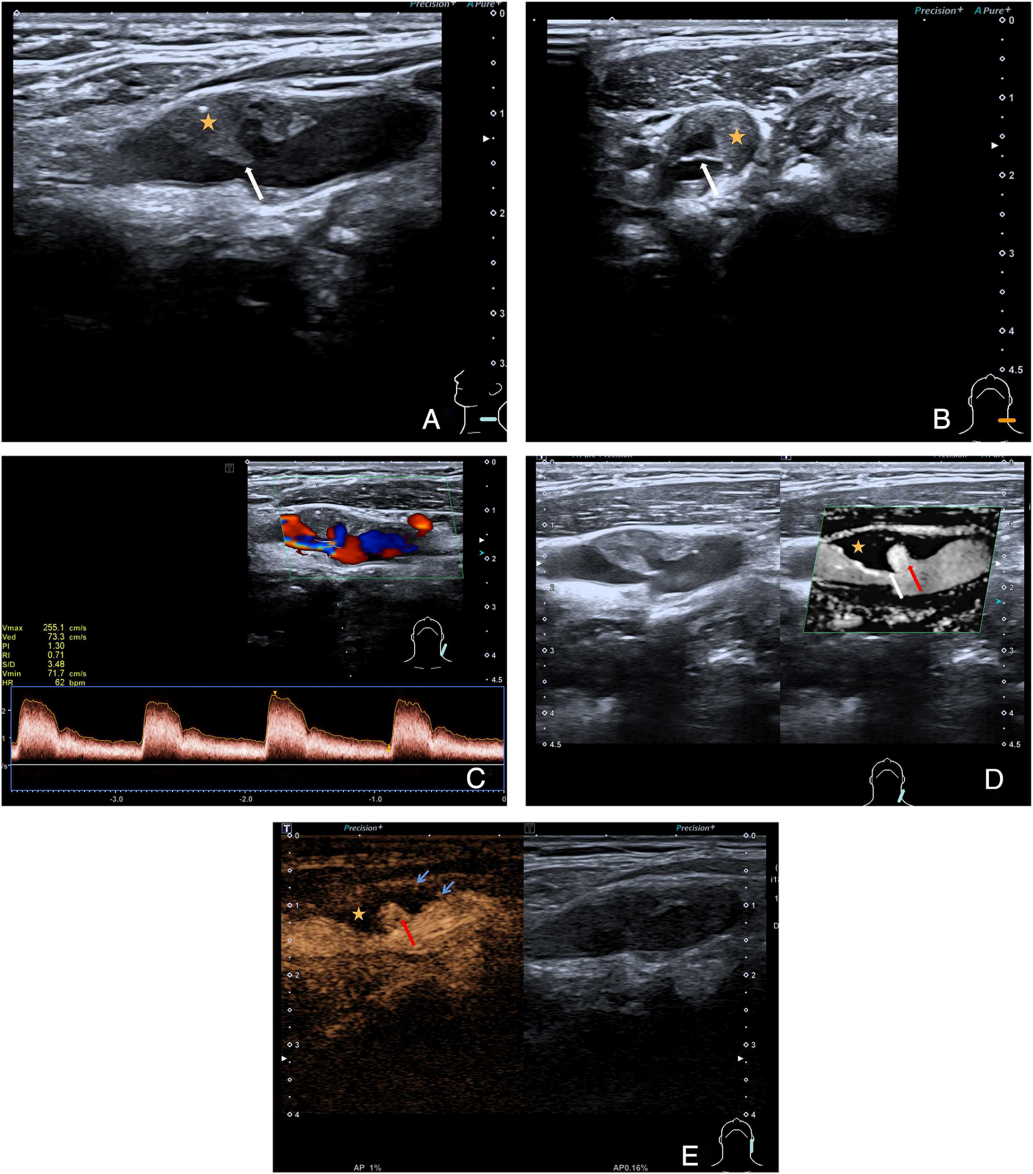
Multimodal ultrasound features of a carotid web (white arrow) with plaque formation (orange star) which locates on the anterior wall of the left carotid artery bifurcation. A B-mode ultrasound shows an uneven isoechoic plaque on the longitudinal plane with a shelf-like structure protruding into the lumen. B A thin membrane-like structure on the cross plane. C Color doppler ultrasound shows swirling blood and severe arterial stenosis. D Superb microvascular imaging reveals triangular endoluminal defect. E Contrast-enhanced ultrasound shows contrast agents entering the cavity (red arrow), with plaque enhancement (grade 1, blue arrows)
